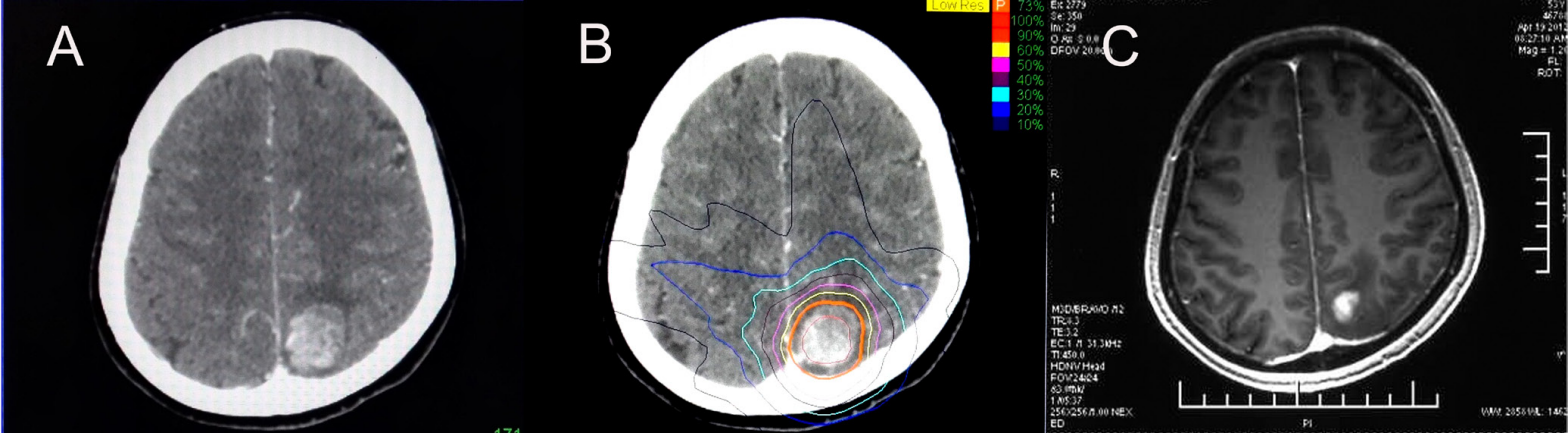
CyberKnife treatment and effects A. CT scan before treatment (February 2012); B. a follow-up in April 2012 showed a significantly smaller lesion.
